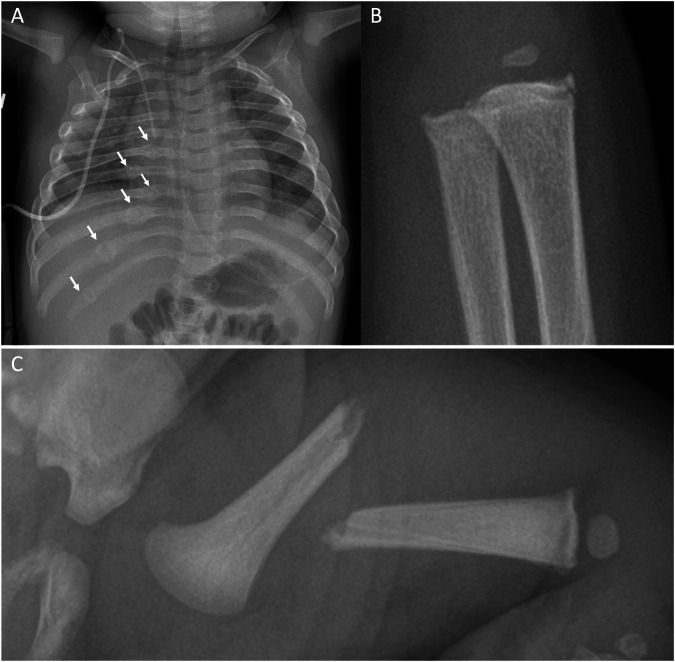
Fractures/presentations typical of inflicted injury. A Anteroposterior (AP) chest radiograph in a 9-month-old girl with no history of trauma, shows healing fractures of the posterior arcs of the right 6th–11th ribs (arrows). B AP radiograph of the left wrist in an 8-month-old boy shows classic metaphyseal fractures of the distal radius and ulna (circles). C AP radiograph of the left femur in a 5-week-old boy shows a displaced and angulated mid-shaft fracture. There is no subperiosteal new bone formation nor is there a callus, consistent with an acute fracture. There was no convincing history of trauma
